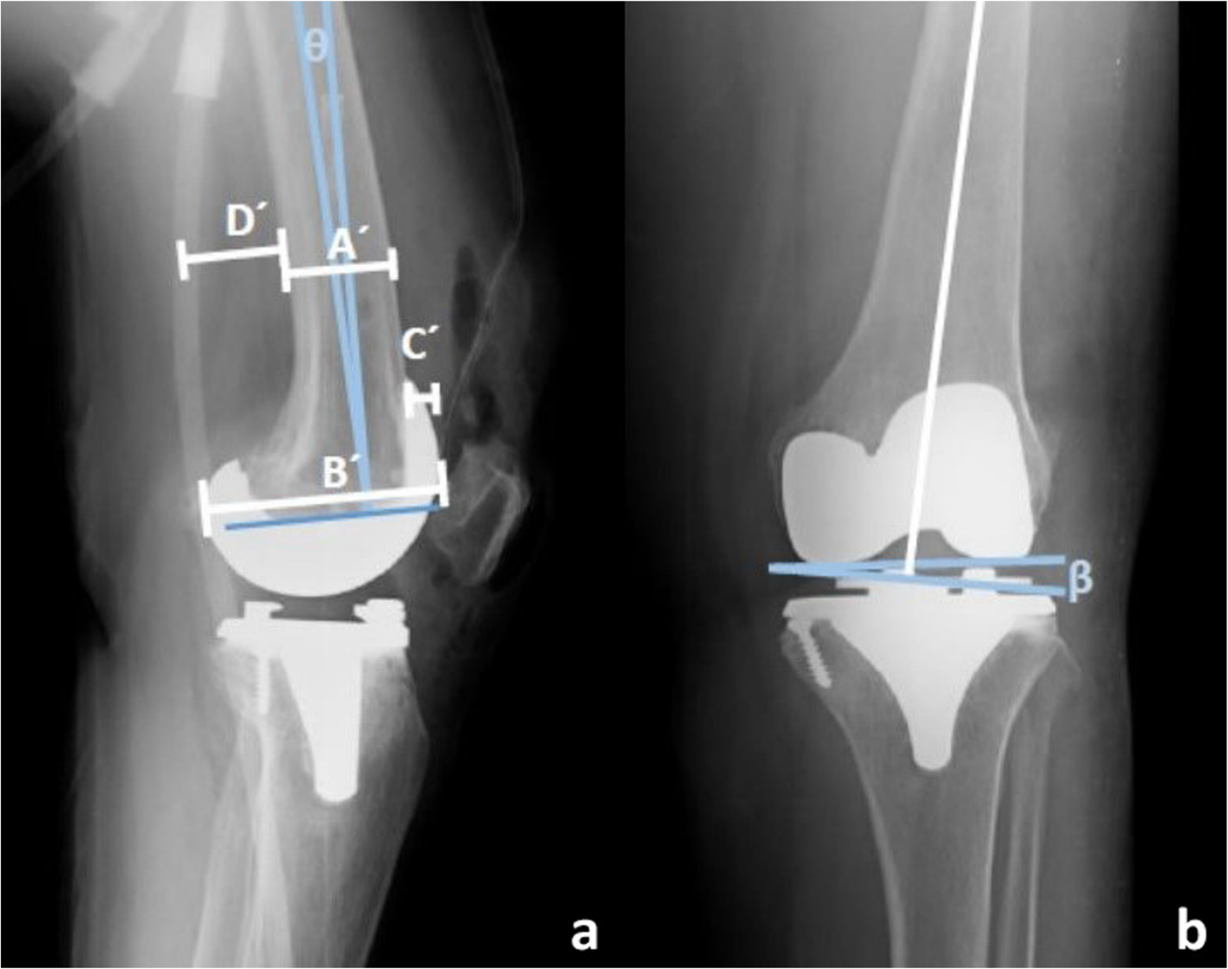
Postoperative radiographic measurements of femoral component. a: Measurement technique of diameter of AP femoral canal (A’), diameter of AP femoral condyle (B’), anterior femoral offset (C’), posterior femoral offset (D’) and femoral component flexion angle (ө) in lateral view. b: Measurement technique of femoral valgus angle (β)
